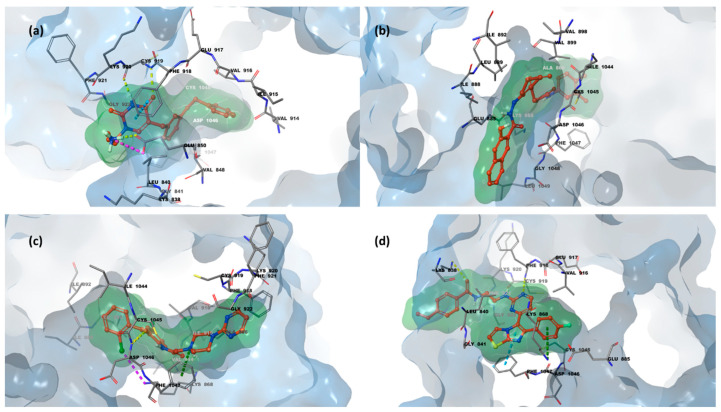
(a) 3D x-ray structure of VEGFR-2 binding pocket in complex with compound 623292; (b) 3D structure of VEGFR-2 in complex with compound 625894; (c) 3D structure of VEGFR-2 in complex with compound 737734; (d) 3D structure of VEGFR-2 in complex with compound 753203. Protein carbon atoms are shown in grey, ligand carbon atoms in red, oxygen atoms in red, nitrogen atoms in blue, and sulfur atoms in yellow.
